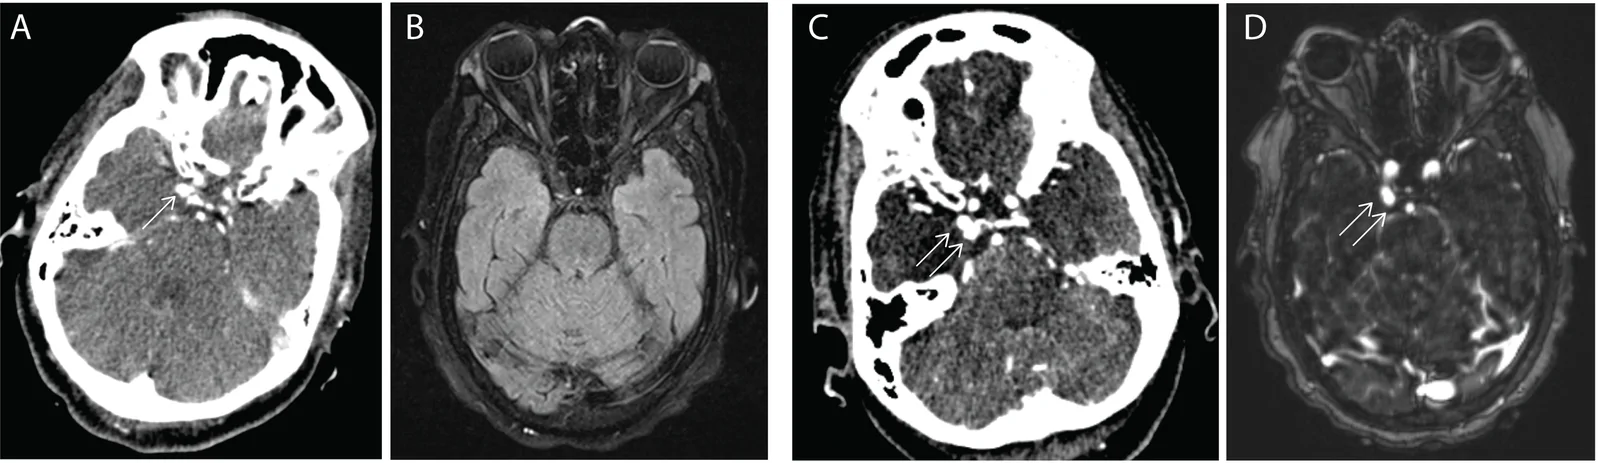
Computed tomography (CT) angiography and MRI angiography showing aneurysms at the posterior communicating artery Axial view of: A) CT angiography at the first admission showing aneurysms (denoted by arrows) at the junction of the posterior communicating artery and the internal carotid artery, and B) MRI brain and orbit at the first admission showing the brain and orbit without any mass lesion. C) Repeat CT angiography and D) MRI angiography at the second admission showing bilobed aneurysms (denoted by arrows) at the junction of the posterior communicating artery and the internal carotid artery.
